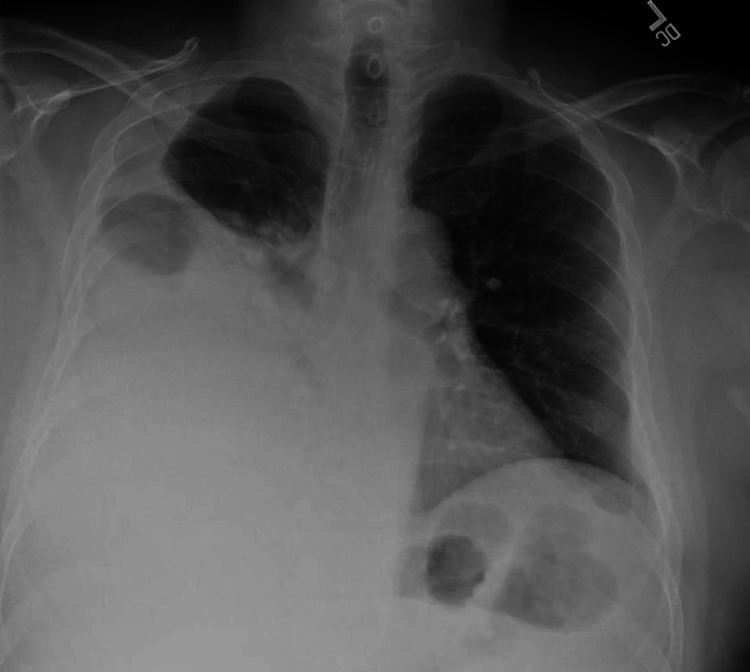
Chest x-ray demonstrating near complete opacification involving the mid to lower right lung along with moderate right side pleural effusion with minimal contralateral shift of the heart to the left side. The left lung is clear.
